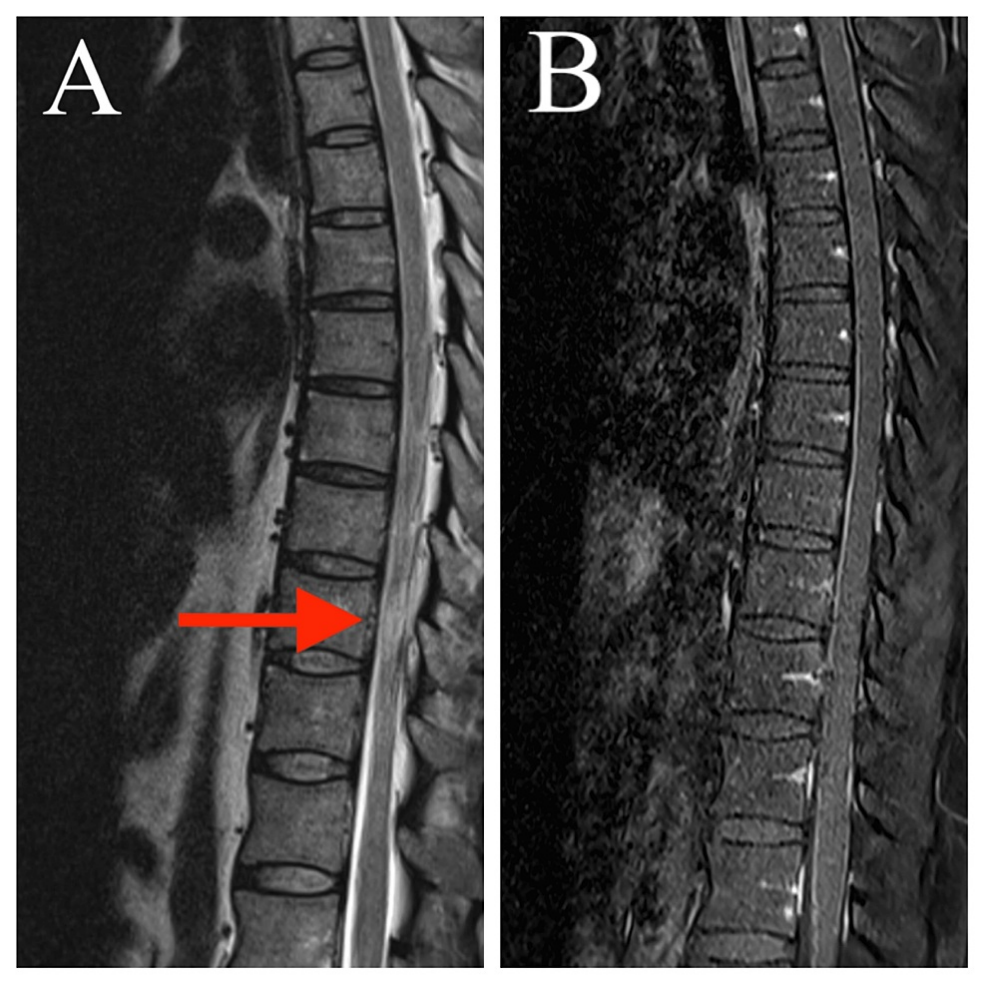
MRI thoracic spine sagittal (A) T2 shows hyperintense signal at T7-T10 cord level and (B) T1 contrast shows no post-contrast enhancement.
